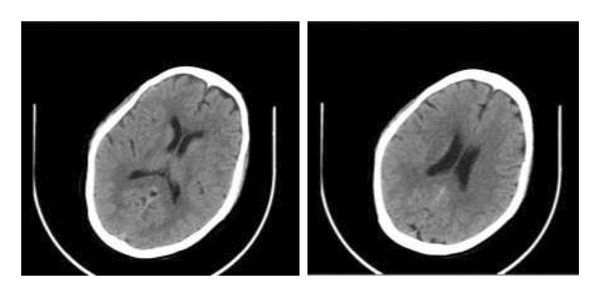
The plain CT brain imaging on the 9th day after initial symptoms.
